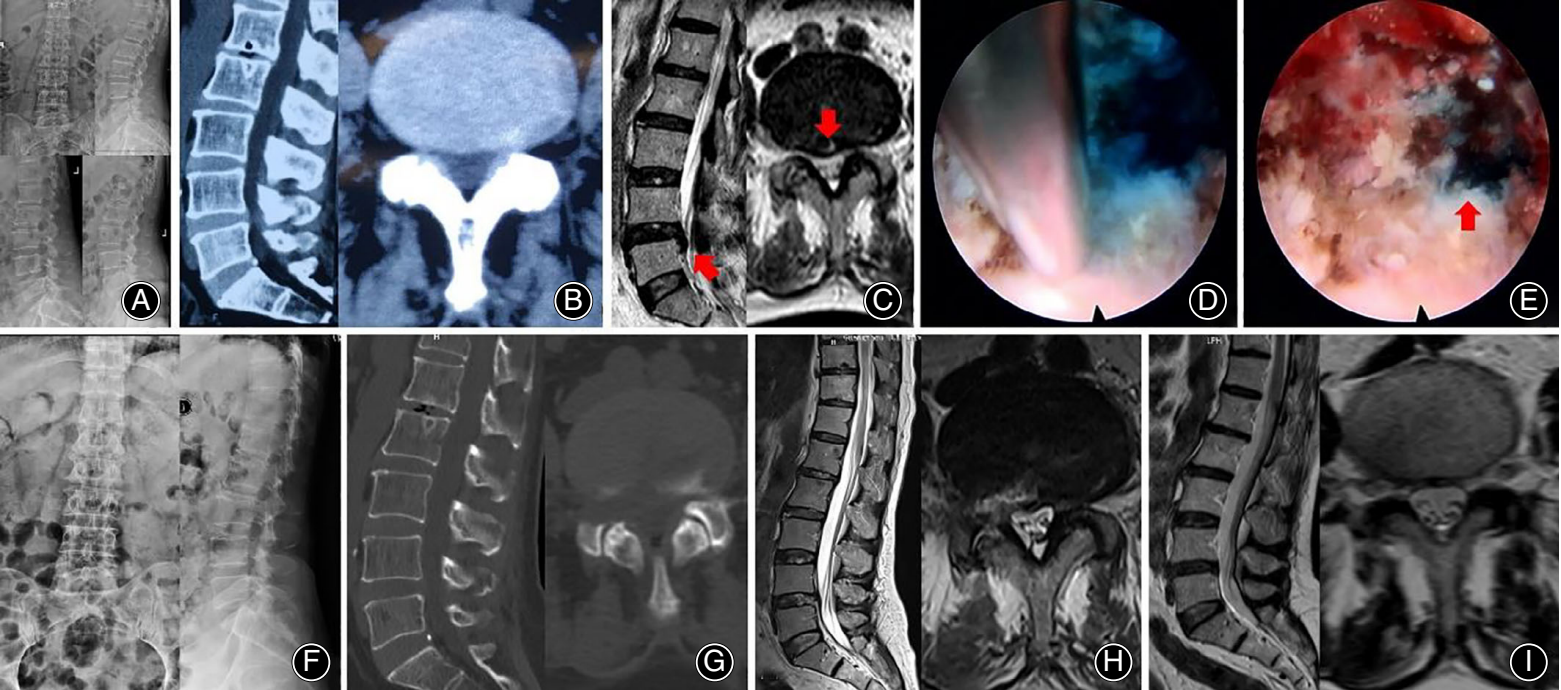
A 58‐year‐old female patient suffered from right lower extremity pain for 3 months and underwent L4‐5 PELD surgery. (A, B, and C) Preoperative lumbar spine X‐ray, CT, and MRI. Her diagnosis was L4‐5 LDH (arrow). (D and E) The long syringe is punctured into the intervertebral disc under the endoscopic view and the methylene blue mixture is injected. The rupture of the annulus fibrosus appears under endoscope. (F, G, and H) Postoperative lumbar spine X‐ray, CT, and MRI. MRI showed the herniated disc has been removed completely. Postoperative ODI value 10% value and VAS score (0 score) were significantly lower than preoperative ODI (48%) and VAS (5 score). (I) Lumbar MRI images at 6 months follow‐up showed annulus fibrosus self‐rehabilitation.
